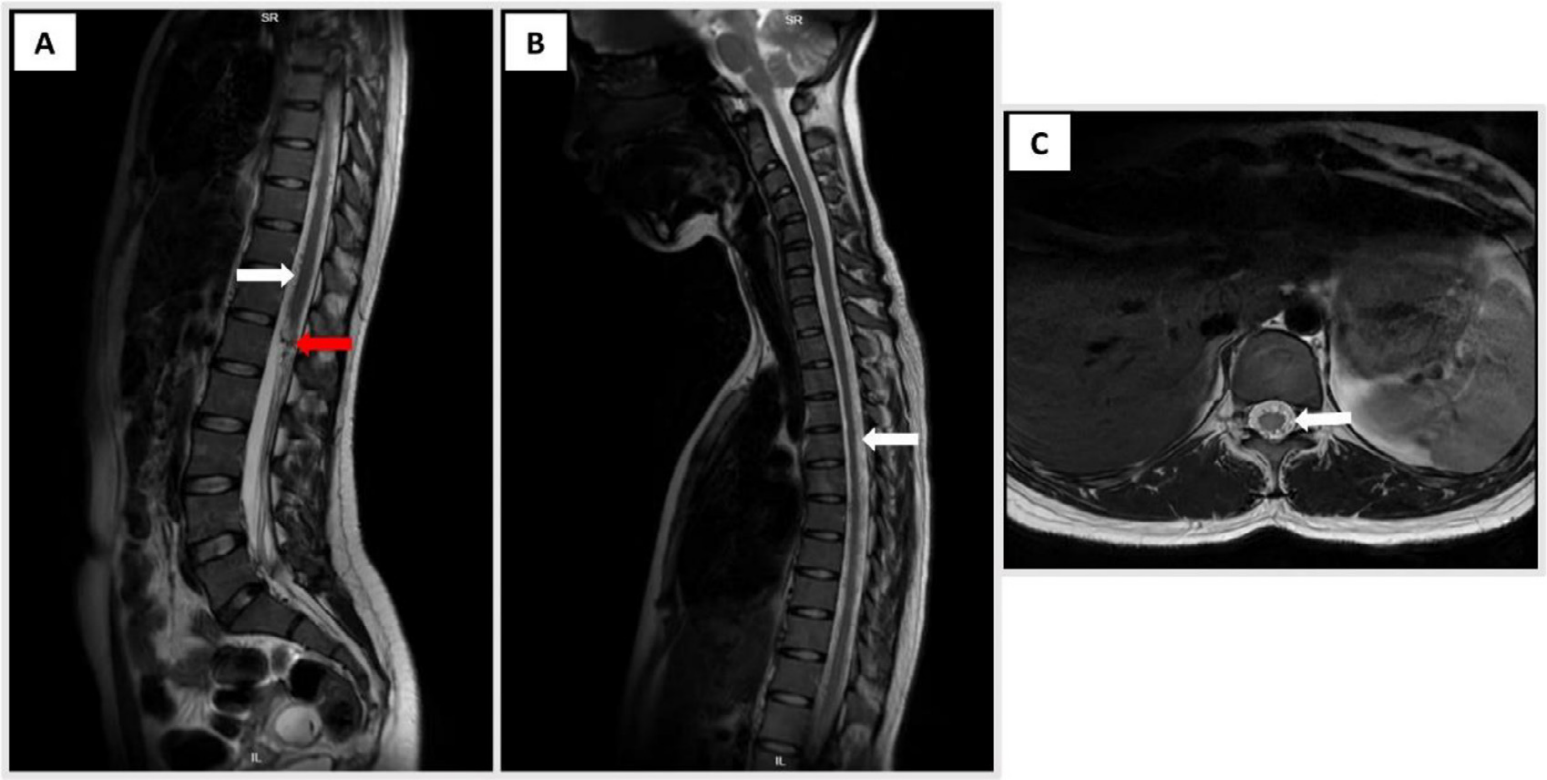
Postembolization MRI. (A) Sagittal T2-weighted MR image of the thoraco-lumbar spine showing a decrease in spinal cord edema, minimal dilated vascular flow voids around the spinal cord (white arrow), and Onyx cast (red arrow). (B) Sagittal T2-weighted MR image of the cervico-thoracic segment showing decreased perimedullary flow void compared to before. (C) Axial T2-weighted MR image showing minimal dilatation of perimedullary vein in thoracal level.
